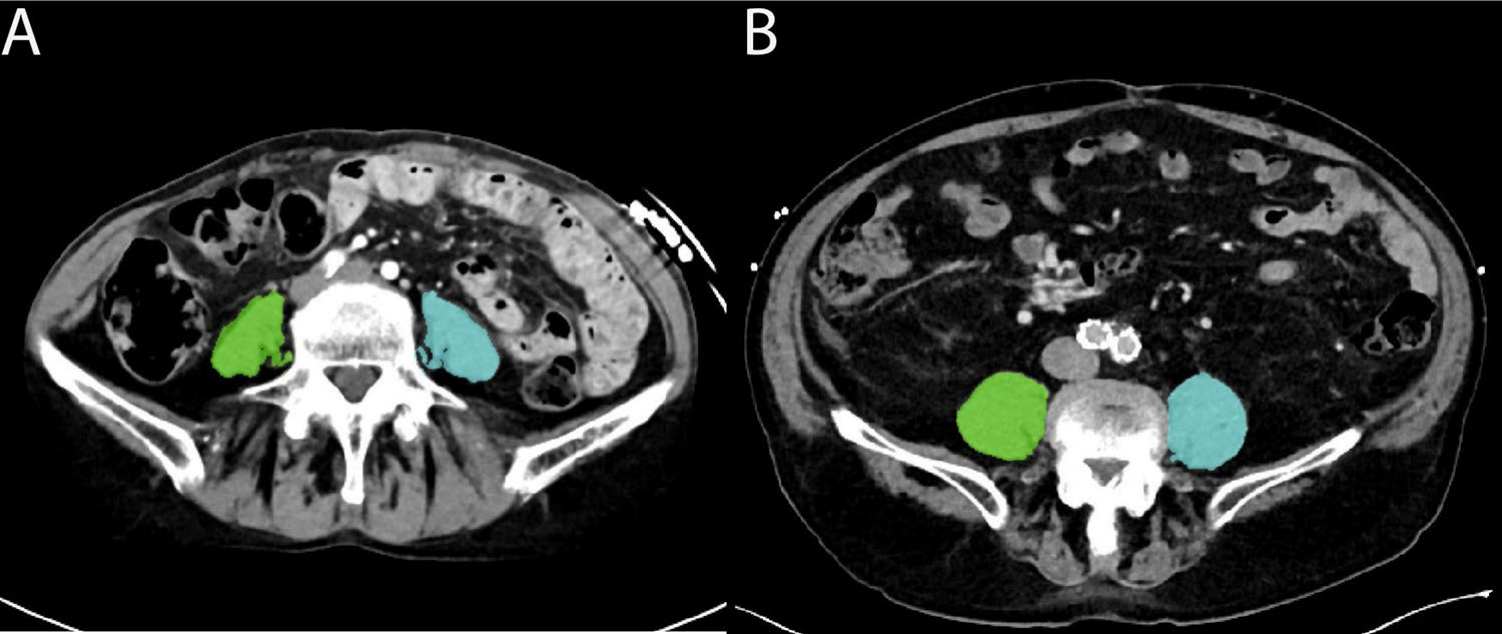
Assessment of psoas muscle area using computed tomography. Axial computed tomography (CT) images showing patients with low psoas muscle area (A) and high psoas muscle area (B)
